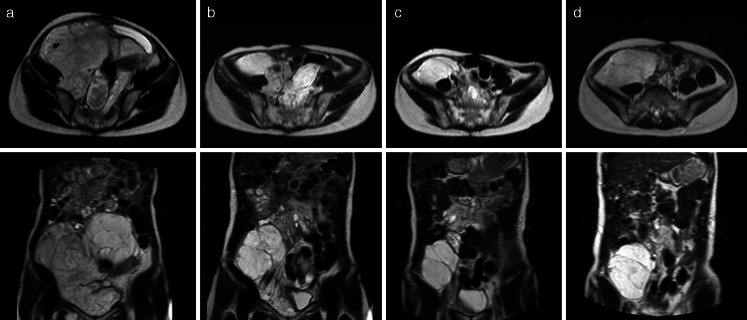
MRI findings of a 21-year-old female patient with a recurrent retroperitoneal myxoid liposarcoma: a baseline, and after b 7 cycles (day 232), c 13 cycles (day 421) and d 19 cycles (day 672) of trabectedin at dose level 2 (1.2 mg/m^2^)
